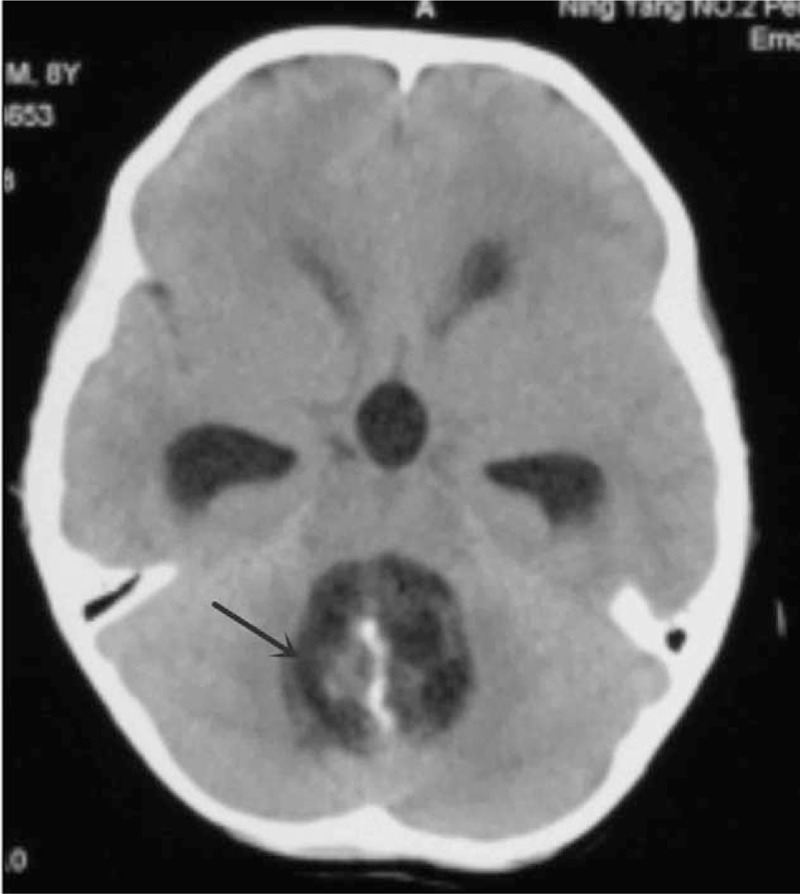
Preoperation CT. CT = computed tomography.
